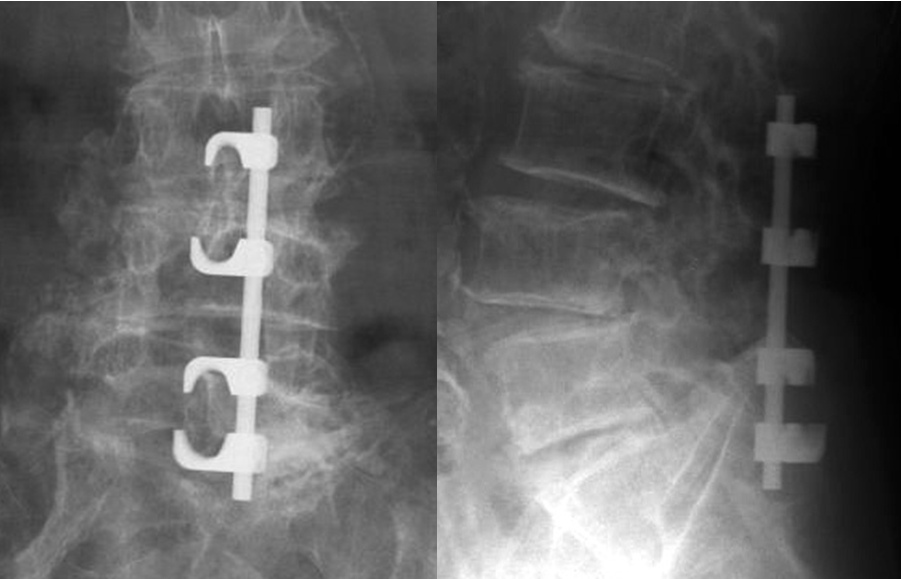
X-rays of a case of two-level fusion
